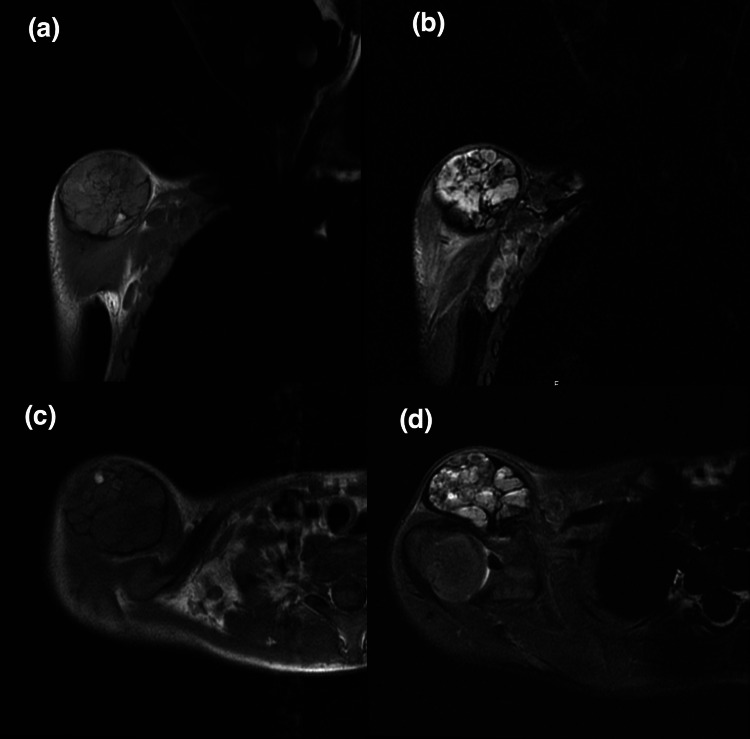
A 6-year-old boy diagnosed with angiomatoid fibrous histiocytoma (case 1): (a) coronal and (c) axial T1-weighted non-fat saturated images. (b) coronal and (d) axial STIR images. A 3.3×2.9×3.1 cm showing multi-speptate cystic lesion in the shoulder. STIR: short T1 Inversion Recovery.
